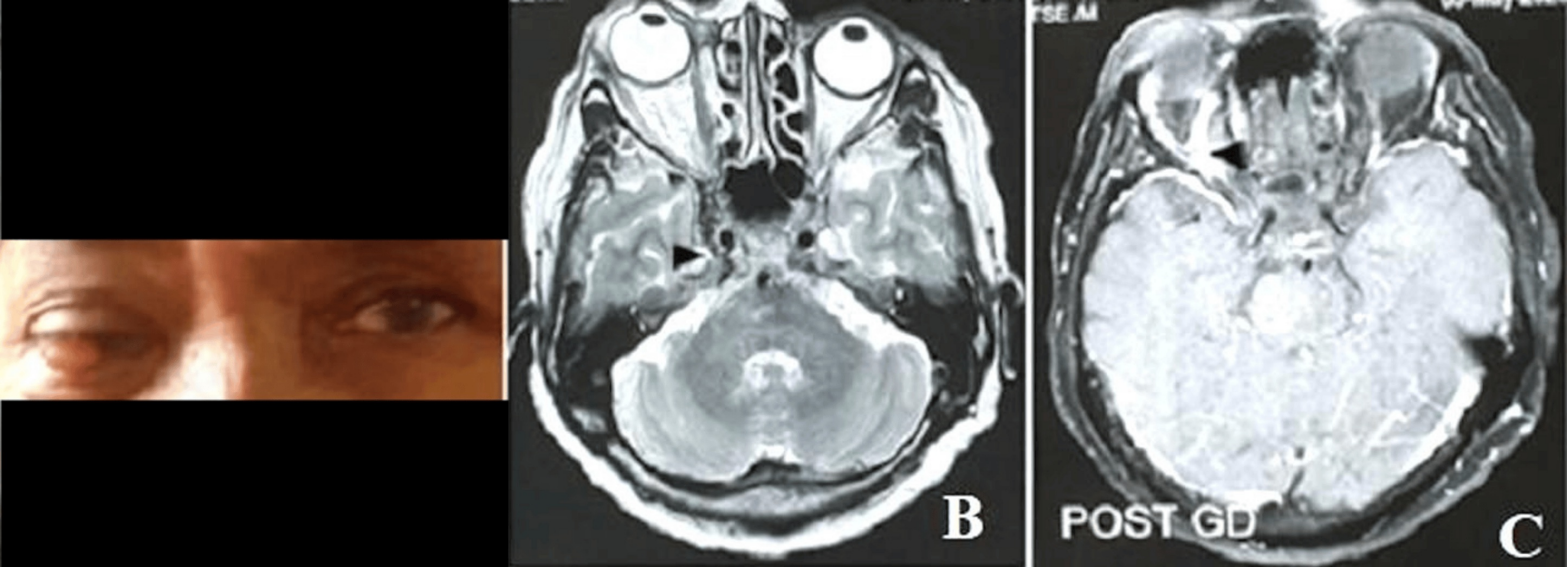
Clinical and MR images of the patient with carotid-cavernous fistula (A) The clinical photograph shows redness and proptosis of the right eye. (B, C) Axial T2-weighted and post-contrast images of the brain showing proptosis of the right eye, bulky right cavernous sinus, and dilated right superior ophthalmic vein. MR: magnetic resonance
